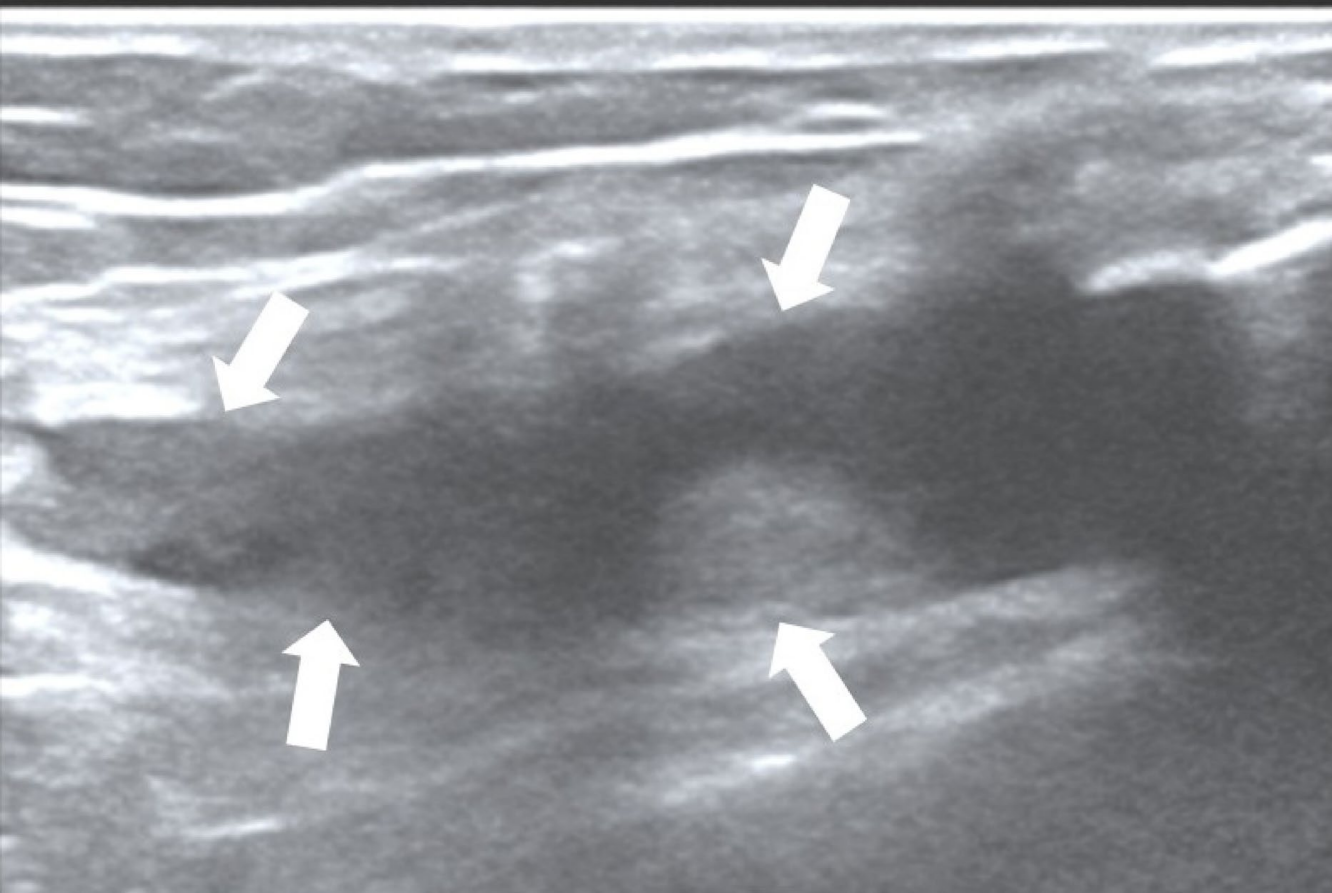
Grey-scale (GS) sonogram. Example of ultrasound imaging showing grade 2 (moderate) GS joint inflammation at the supra-patellar recess of the knee (white arrows pointing towards synovial hypertrophy) in a patient with rheumatoid arthritis.
